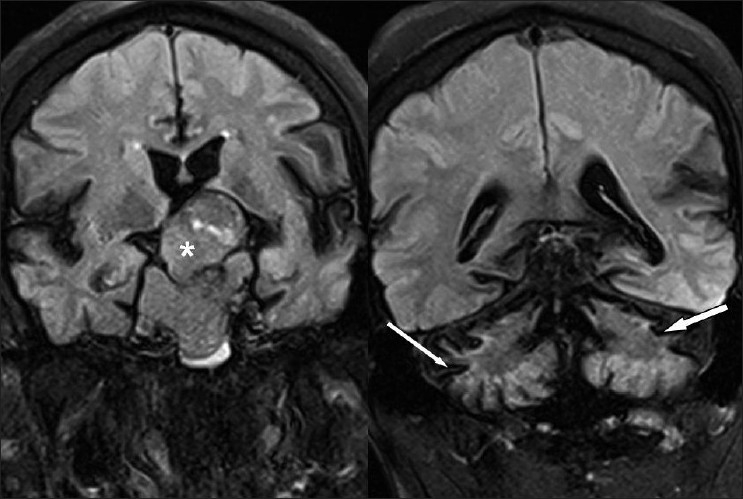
Coronal T2 FLAIR images showing large heterogeneous sellar-suprasellar mass (asterisk) and low signal along and cerebellar surfaces (white arrows)
